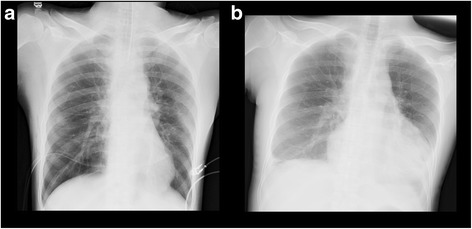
Postoperative chest radiography on the spine position: a immediately after the operation and b on the postoperative day 11. The cardiothoracic ratio was increased from 46.0 to 64.2%
